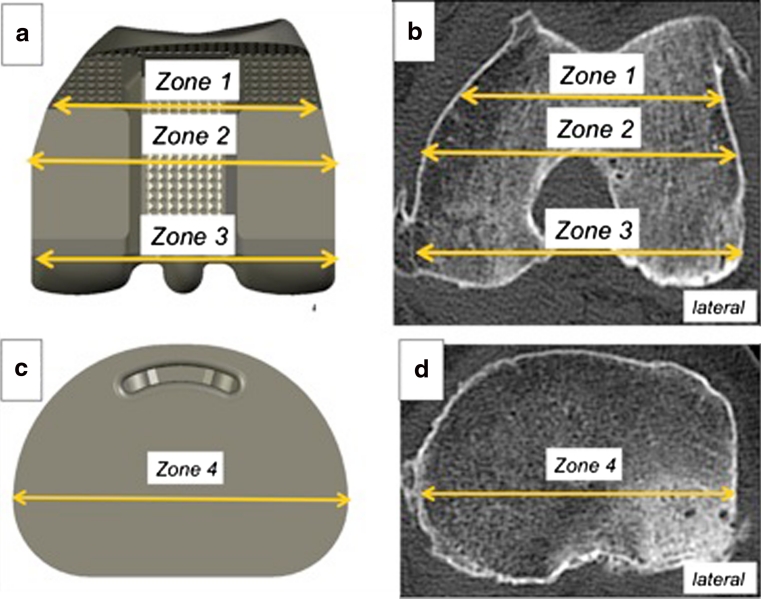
Three reference zones were defined on the femoral implant a zone 1, corresponding to the posterior part of the anterior chamfer, was located at a variable distance from the posterior bicondylar line (BCL) depending on the implant size (39.4–48.5 mm; see Appendix in ESM). Zone 2 was located at a variable distance from the posterior bicondylar line (BCL) depending on the implant size (26–36 mm; see Appendix in ESM), but was directly posterior to the point where the implant began to narrow. Zone 3 corresponds to the posterior condylar bone cut, situated 10 mm from the BCL. On the CT scan b, the analysis was done on the axial cut located at the level of the distal femoral cut made at the time of surgery (10 mm from the most distal point of the medial condyle). The bone dimensions corresponding to the three zones defined were measured: zone 1, 10 mm from the BCL, zone 2 and zone 3, at the distance corresponding to the size of the implanted prosthesis. On the tibia, the mediolateral dimension (zone 4) was used as the reference (c). On the CT scan, the measurement was taken on the axial cut located at the bone cut made at the time of surgery (d). The transverse, mediolateral dimension was measured
